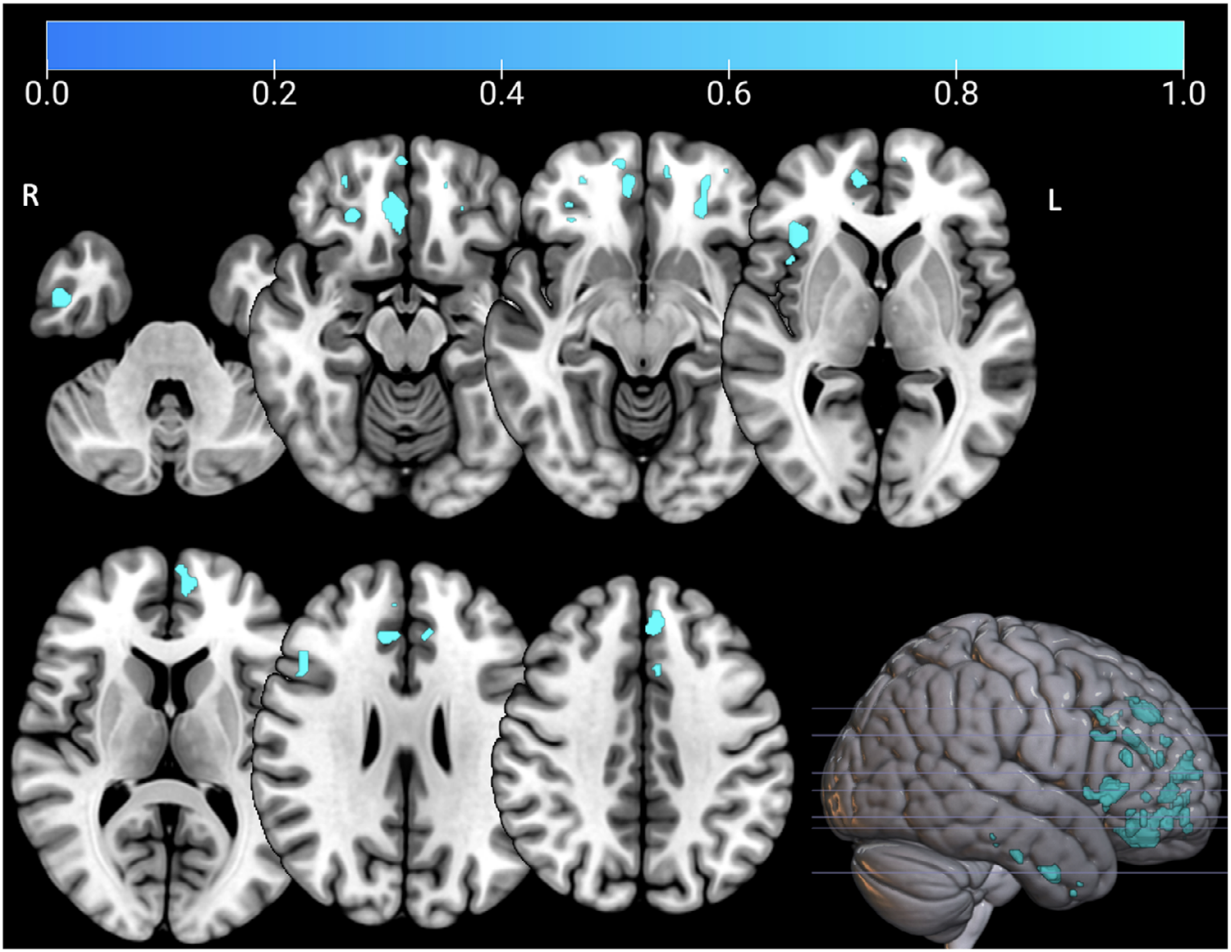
Neuroanatomical correlates of performance on the Dutch version of the Social Norms Questionnaire total score. Results are shown on a study‐specific T1‐weighted magnetic resonance imaging template in Montreal Neurological Institute space. Results are corrected for family‐wise error (*P* < 0.05).
